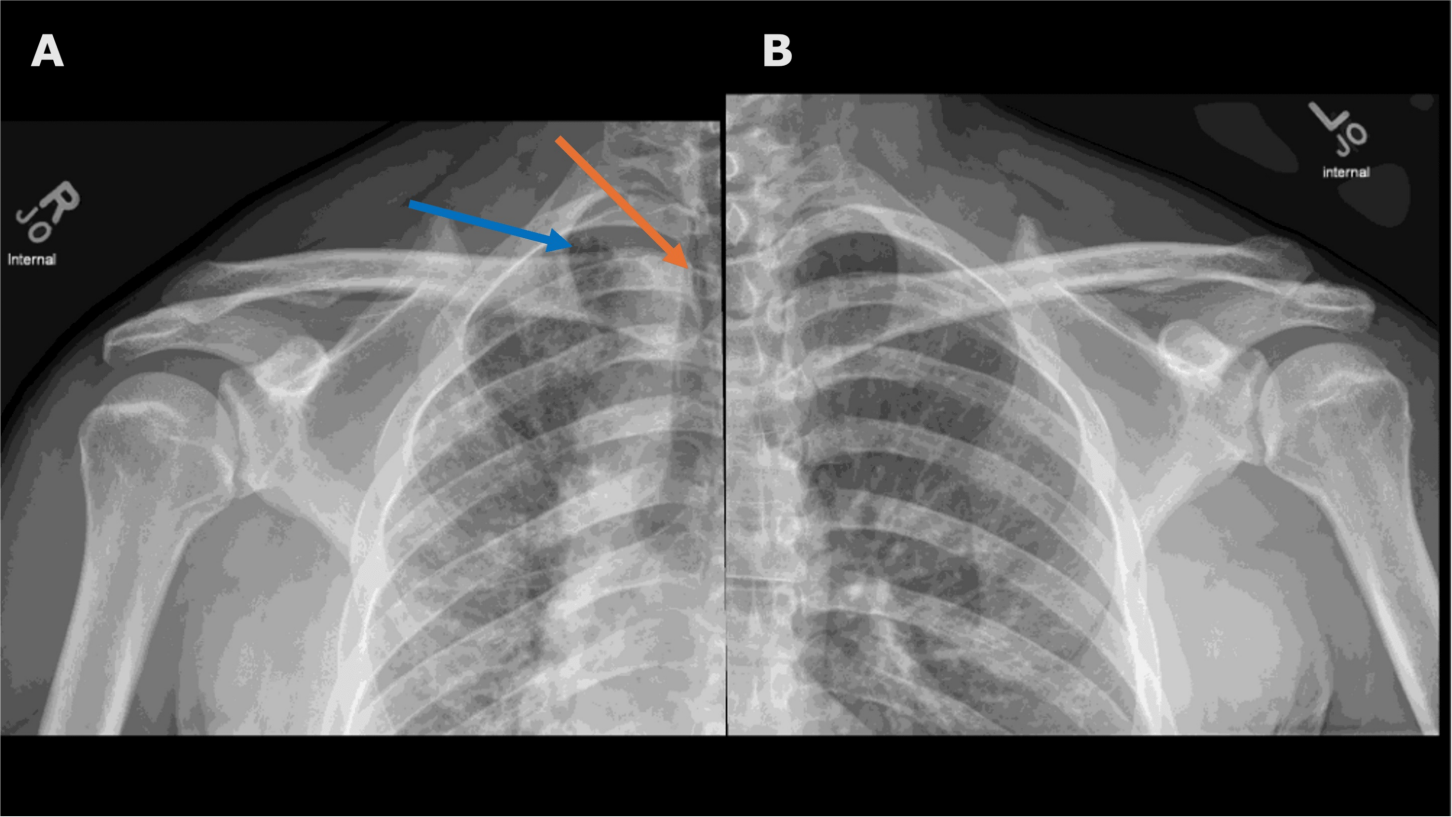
Bilateral shoulder X-rays evaluating causes for shoulder pain. The bilateral shoulder radiographs reveal a decrease in volume in the right lung, evidenced by a shift in the mediastinum and trachea (orange arrow). Additionally, there is a noticeable enhancement of the interstitial patterns and reticulation in the right lung (left image, A), especially in the upper lung (blue arrow). The left lung appears normal on the radiograph (right image, B). Bilateral shoulder pain was diagnosed as mild to moderate osteoarthritis.
